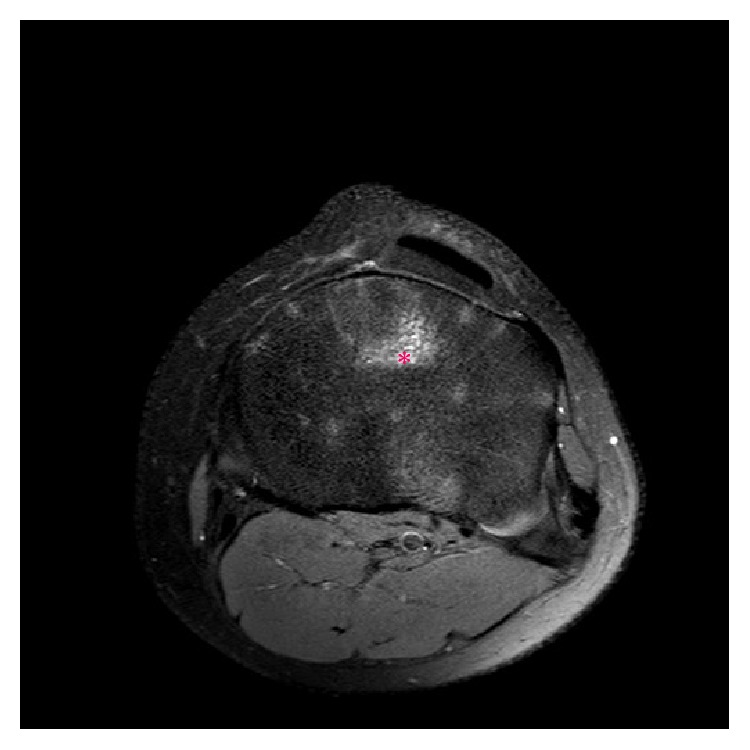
A 13-year-3-month-old female with FOPE zones at both proximal tibiae. Findings: axial proton density fat-saturated image at the level of the proximal tibia physis shows the periphyseal edema located at the anterocentral aspect of the central physis (asterisks). Technique: axial PD fat-saturated MRI (TR = 2,843.2, TE = 30), ST = 3 mm, spacing = 3.3 mm, FOV = 15 cm, and matrix = 376 × 297.
